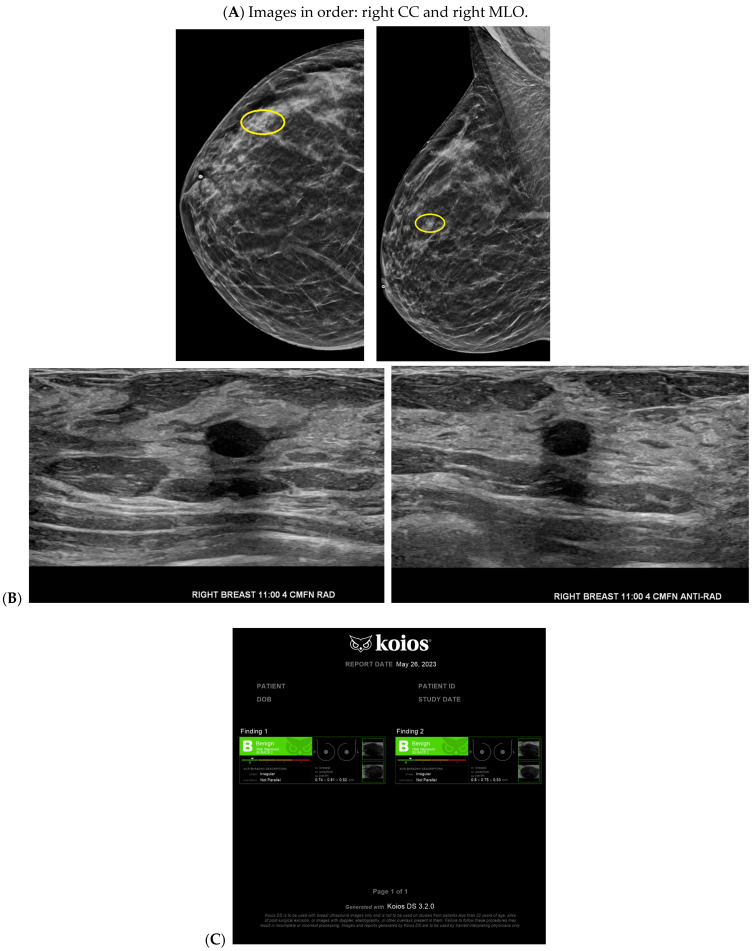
A benign finding appropriately classified as benign by artificial intelligence (AI): The patient initially presented for a bilateral screening mammogram and a bilateral screening breast ultrasound. A mammogram revealed benign dystrophic calcifications in the upper outer quadrant of the right breast (A). Correlating with findings on the mammogram, ultrasound revealed a complicated cyst showing posterior acoustic shadowing consistent with fat necrosis (B). The AI program Koios recognized this mass as “Benign” (C). Images obtained from the Icahn School of Medicine at Mount Sinai.
